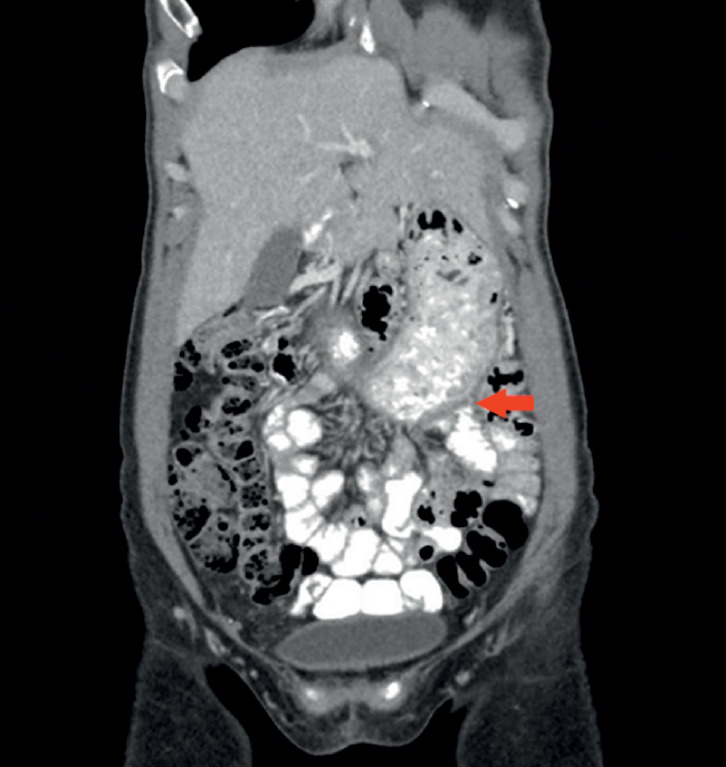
Abdominal computed tomography scan with oral contrast revealed a patent gastroenterostomy anastomosis after removal of the lumen-apposing metal stent.
